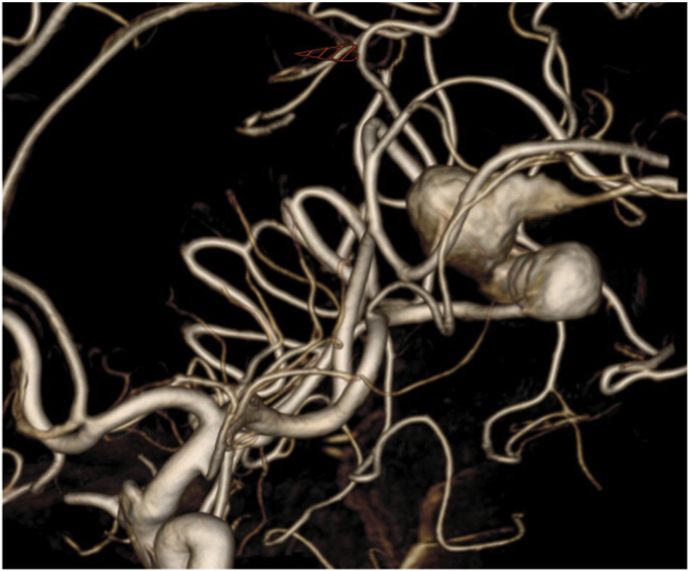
Diagnostic Images of Case #1*. A) T2 weighted brain MRI shows a large distal middle cerebral artery aneurysm with perilesional edema. B Digital Subtraction Angiography; 3D Reconstruction of internal carotid artery injection confirming the fusiform lobulated aneurysm and showing the exiting M3 branch.
